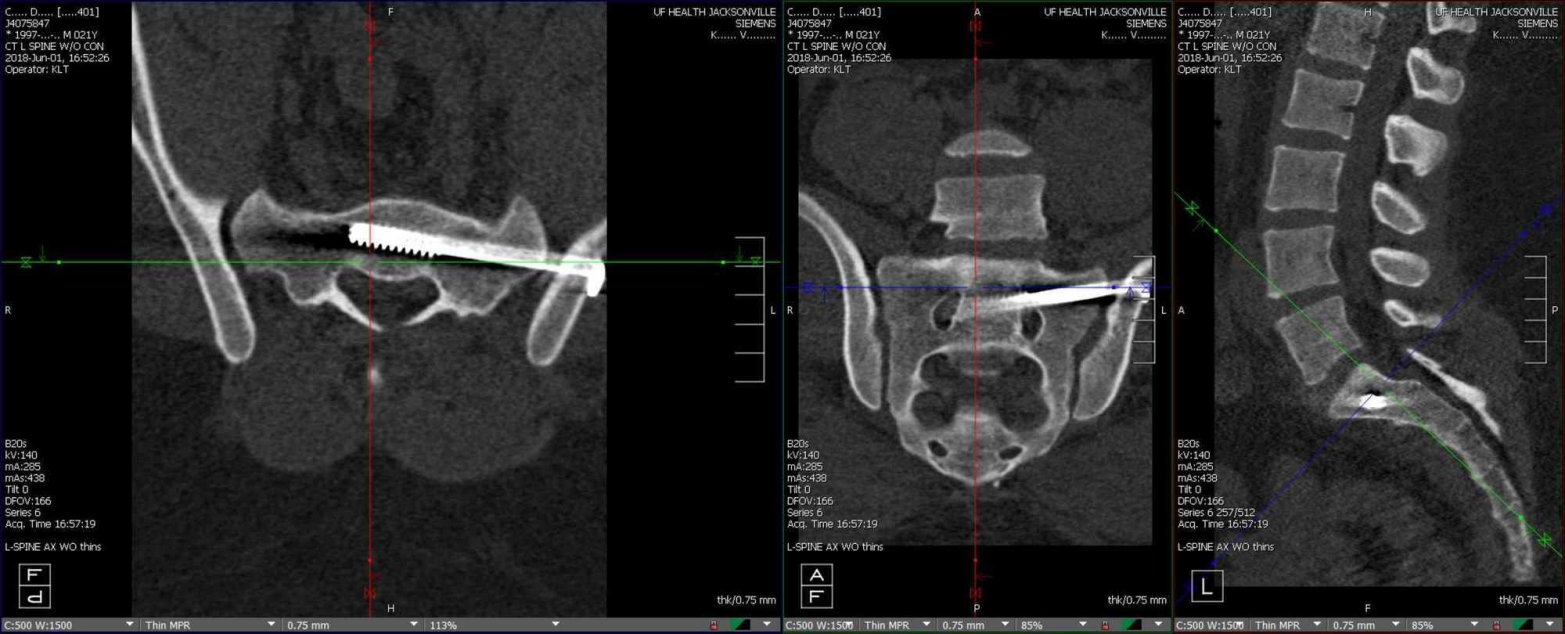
Post sacroiliac stabilization Axial (left), coronal (center), and sagittal (right) CT images revealing post insertion of the left sacroiliac (SI) screw. The SI screw appears to occupy the sacral vertebral body.
